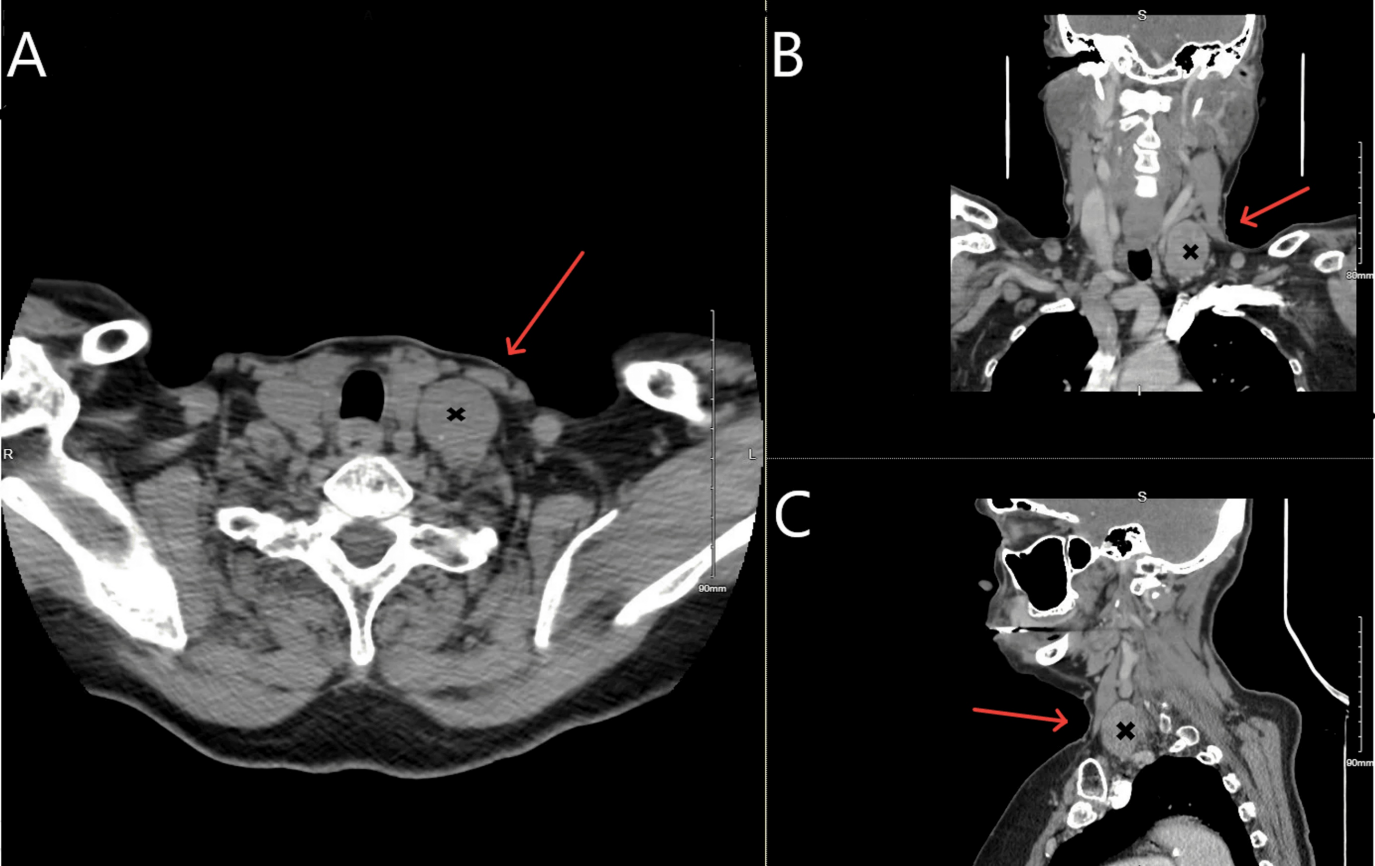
A CT scan of soft tissue neck showing a left neck mass labeled 'X', measuring 4.1 x 2.6 x 3.0 cm in size (red arrows pointing the mass) Panel A showing the axial section, Panel B showing the coronal section, and Panel C showing the sagittal section of the CT soft tissue neck.
